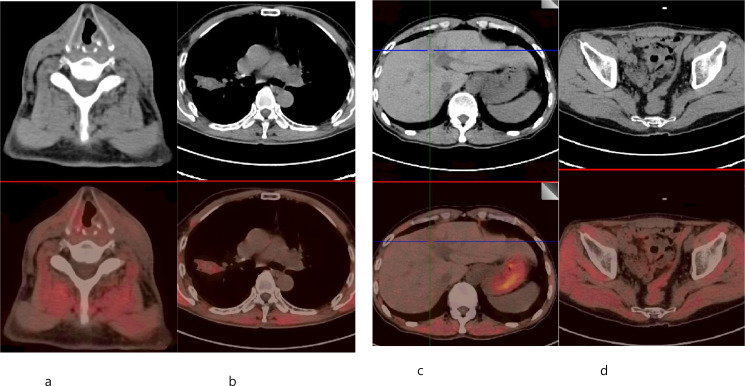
PET/CT images of the patient after treatment. (a) PET/CT shows that the thyroid lesion has almost completely response. (b) PET/CT shows that the lung lesion has almost completely response. (c) PET/CT shows that the liver lesion has almost completely response. (d) PET/CT shows that the pelvic lesion has almost completely response.
